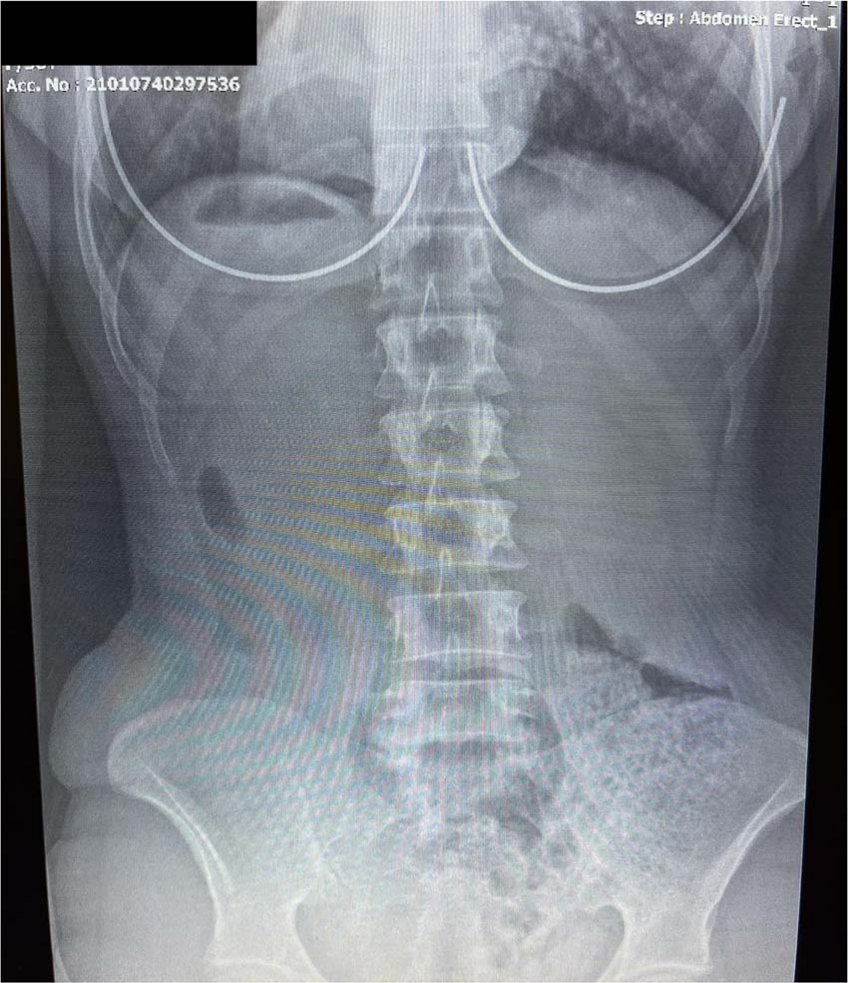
Erect Abdominal Radiograph: Abdominal X-ray (erect view) demonstrating a right-sided gastric air bubble, a hallmark feature of situs inversus totalis. This imaging finding, when correlated with dextrocardia, supports the diagnosis of Kartagener syndrome.
